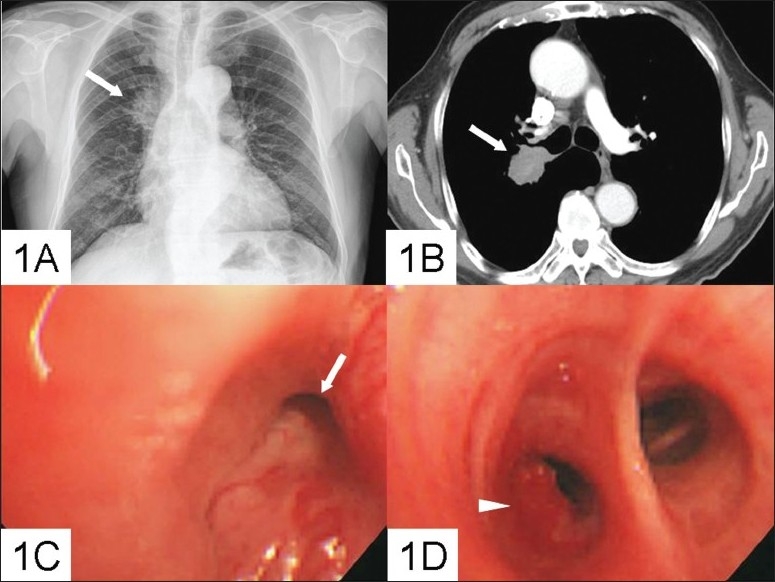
Chest radiograph showing a mass lesion on the right upper lobe (a). The contrast-enhanced CT of the chest shows a right upper lobe soft-tissue mass with encasement of the bronchus, mediastinum invasion and an enlarged right hilar lymph node (b). The bronchoscopic image shows extensive submucosal and lymphangitic infiltration with partial obstruction of the right upper lobe orifice (c) and one whitish, keratinized tumor, easy to bleed on touch and partially occluding the RB7 bronchus lumen (d).
